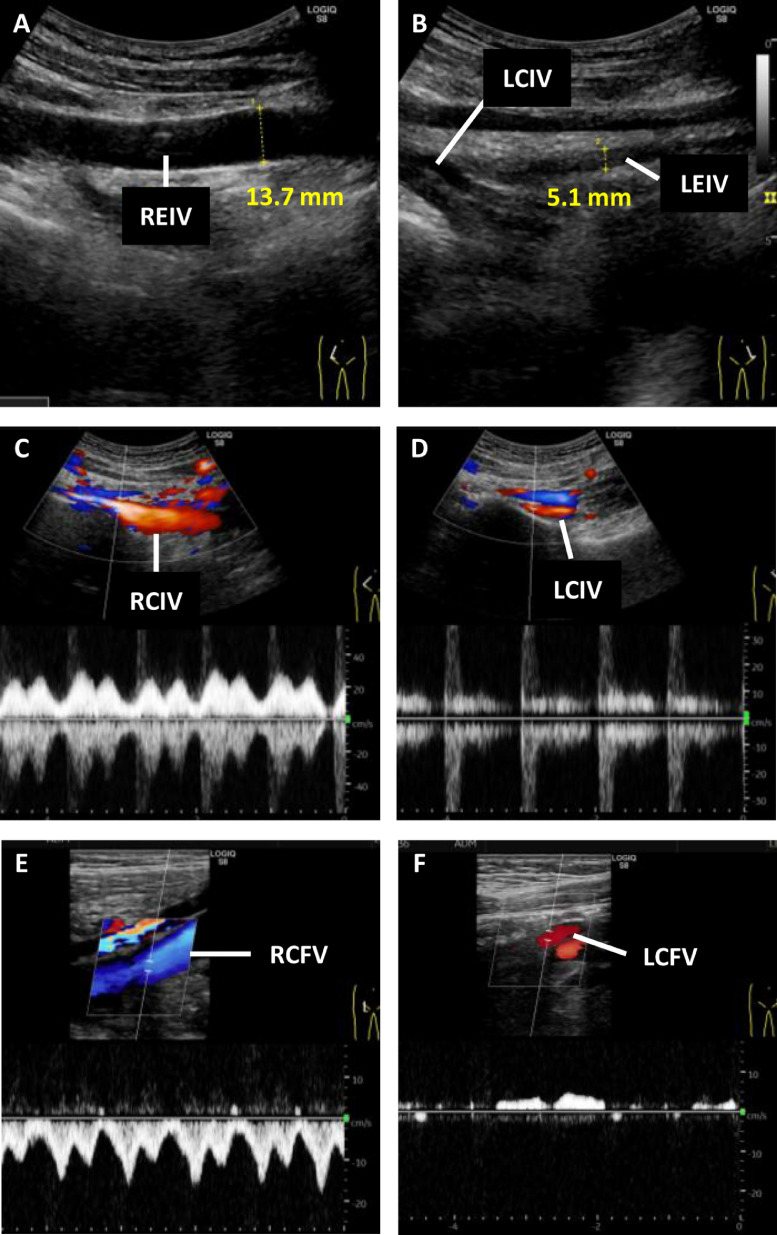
Duplex ultrasonography after resolution of deep vein thrombosis. (A and B) Transversal scan demonstrating that the diameter of the left external iliac vein (LEIV) is smaller than that of the right EIV (REIV). (C and D) Color Doppler ultrasound. The right common iliac vein (RCIV) shows normal phasic flow, while the left CIV (LCIV) shows a lack of phasic flow immediately distal to the site of compression by the right common iliac artery. (E and F) There is no venous reflux in the right common femoral vein (RCFV), whereas the left CFV (LCFV) shows reflux Doppler flow due to hemodynamic changes resulting from compression.
